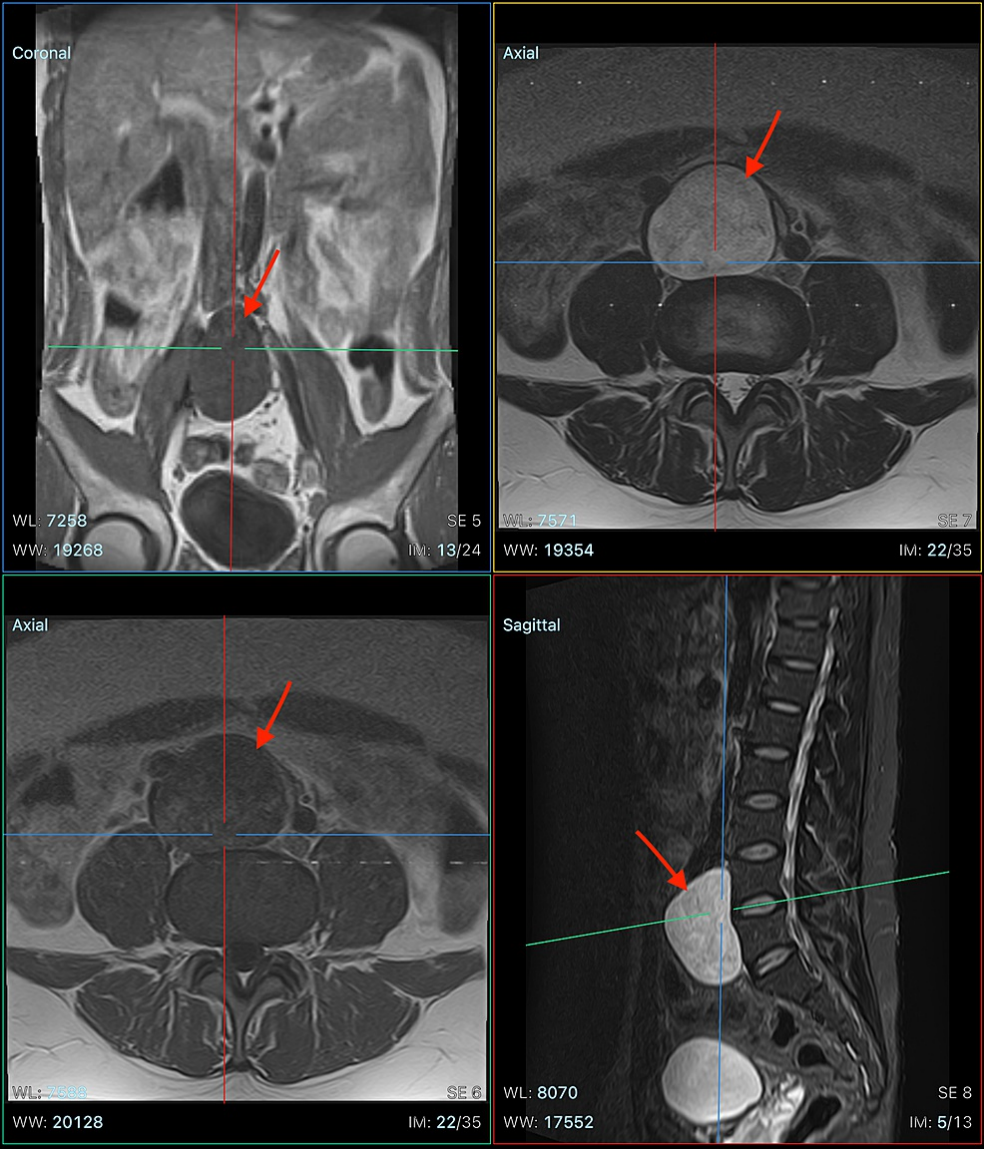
MRI lumbosacral spine showing a retroperitoneal mass isolate from spinal cord structure in coronal (top left), axial (top right, bottom left), and sagittal (bottom right) views. MRI: magnetic resonance imaging
